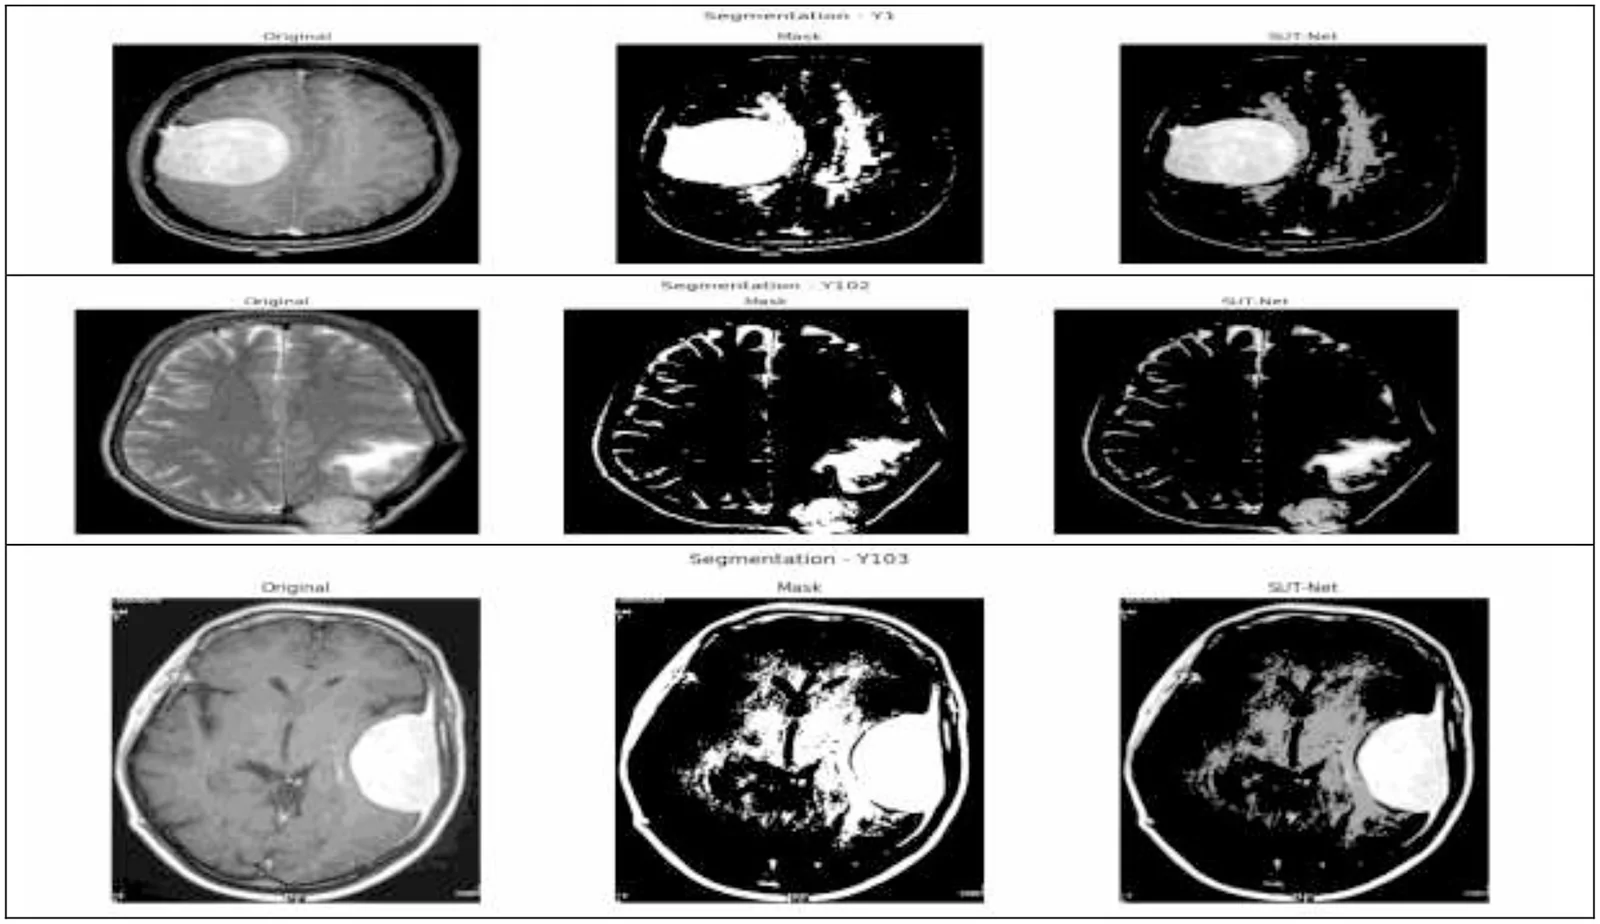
Output of proposed SUT-Net for CT dataset.
